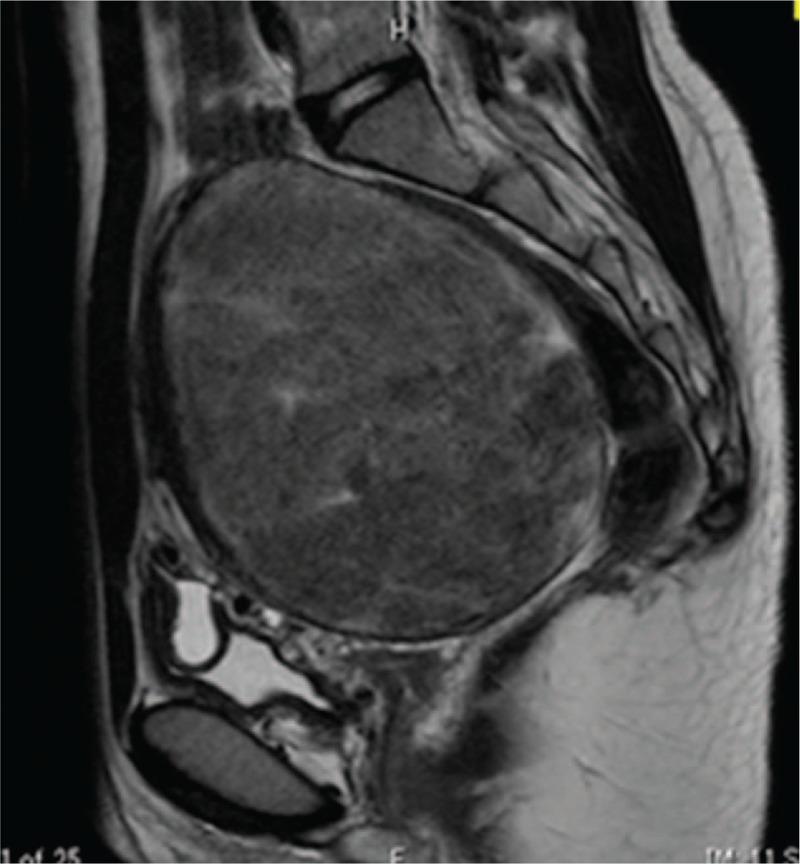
Magnetic resonance imaging (sagittal plane) revealed the solid mass to be within the uterine posterior wall, measuring 11 cm. There was no denaturation in the uterine tumor nor any suggestions of malignancy. It was suspected of being an intramural leiomyoma.
